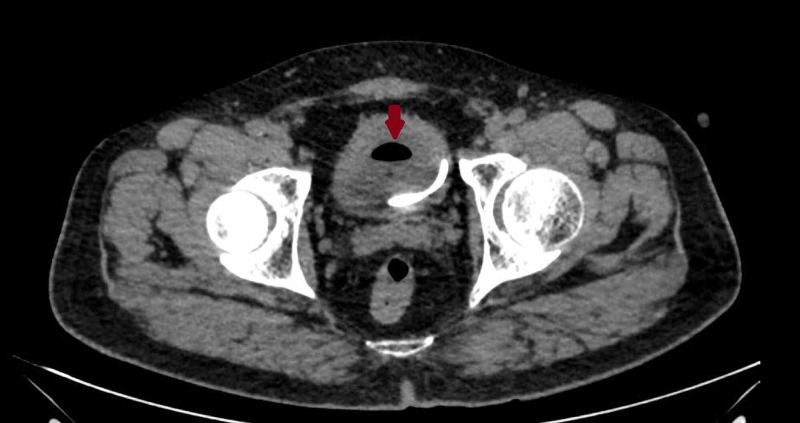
Axial section of CT abdomen revealed the presence of air in the bladder (red arrow) and the distal end of the DJ stent.
